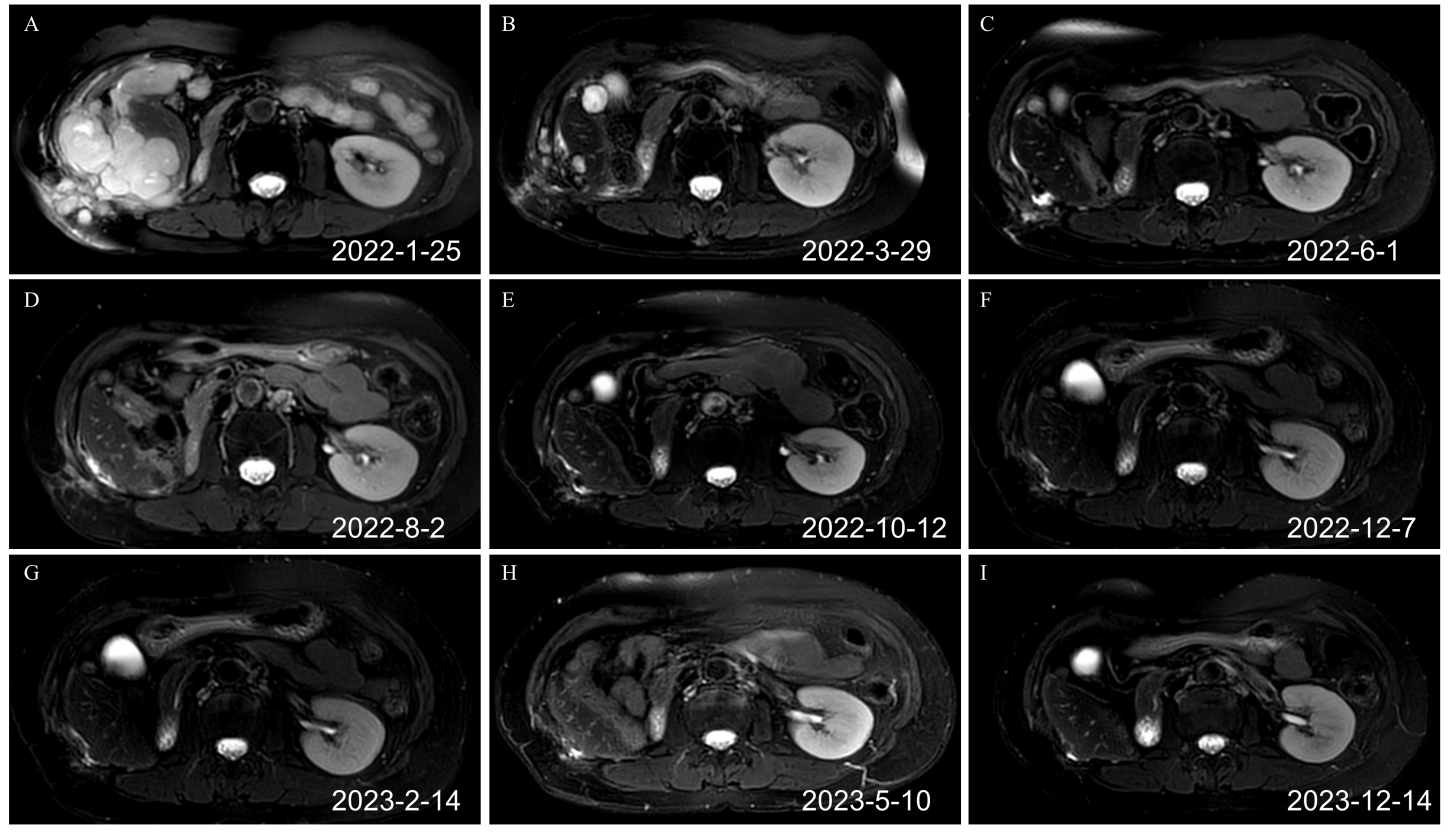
Renal MRI T2 images before and after the combination of toripalimab and anlotinib (axial plane). (A) Recurrence after the second operation before the immunotherapy. Tumors were detected in the liver, abdominal cavity, and abdominal wall, which had fused into one piece (February 25, 2022). (B) After three cycles of targeted immunotherapy, most tumors of the liver, the abdominal cavity, and the abdominal wall disappeared (March 29, 2022). (C) After six cycles of targeted immunotherapy, the tumors of the liver, the abdominal cavity, and the abdominal wall basically disappeared (June 1, 2022). (D) After nine cycles of targeted immunotherapy, the tumors of the liver, the abdominal cavity, and the abdominal wall basically disappeared (August 2, 2022). (E) After 12 cycles of targeted immunotherapy (October 12, 2022). (F) After 14 cycles of targeted immunotherapy (December 7, 2022). (G) After 16 cycles of targeted immunotherapy (February 14, 2023). (H) After 19 cycles of targeted immunotherapy (May 10, 2023). (I) Renal MRI image on December 14, 2023. MRI, magnetic resonance imaging.
